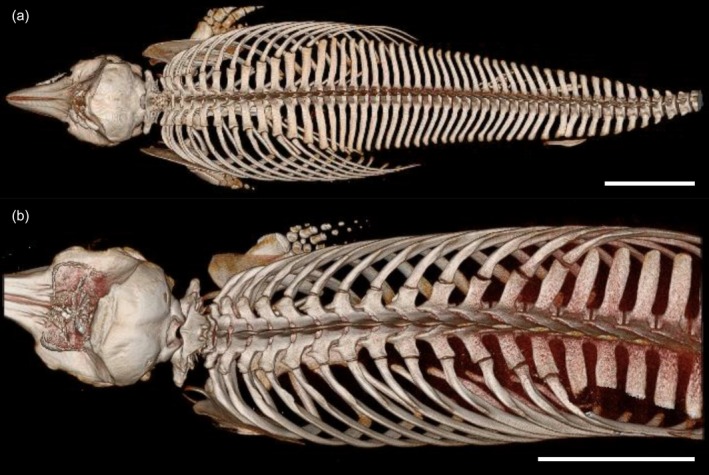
Representation of the skeleton up to the (a) caudal vertebrae of hourglass dolphin (*C. cruciger*) KS20‐20Lc and (b) lumbar vertebrae of spectacled porpoise (*P. dioptrica*) KS20‐07Pd, as displayed by 3D rendering of the CT scans. In (B) there are also hyperintense areas at the level of the nasal sacs, most likely due to the presence of sand. In addition, the left lumbar transverse processes appear truncated, which we confirm is a scanning artifact. Scale bar = 20 cm.
